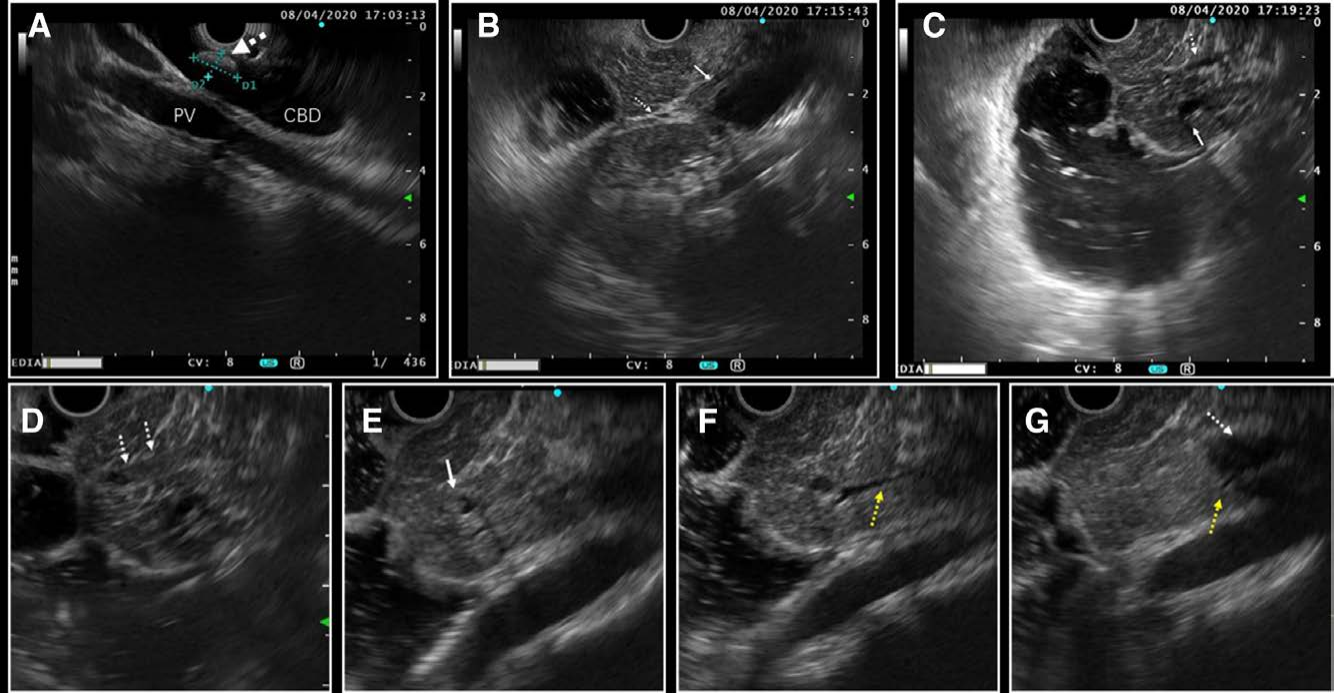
Intraoperative EUS examination during ERCP. (A) Sphincter of Oddi scan showing dilation of the common bile duct with a common bile duct stone (dashed arrow). (B) Duodenal bulb scan revealing dilation of the mid-segment of the common bile duct (arrow) and stenosis in the pancreatic segment (dashed arrow). (C) Local dilation of the main pancreatic duct (arrow) with visibility of the accessory pancreatic duct (dashed arrow). (D) Isoechoic material within the accessory pancreatic duct (dashed arrow). (E) Isoechoic material within the main pancreatic duct (arrow). (F) Branch pancreatic ducts (yellow dashed arrows). (G) Pancreatic duct branch continuous with the common bile duct (yellow dashed arrow), indicated by the white dashed arrow pointing to the common bile duct. ERCP = endoscopic retrograde cholangiopancreatography, EUS = endoscopic ultrasound.
